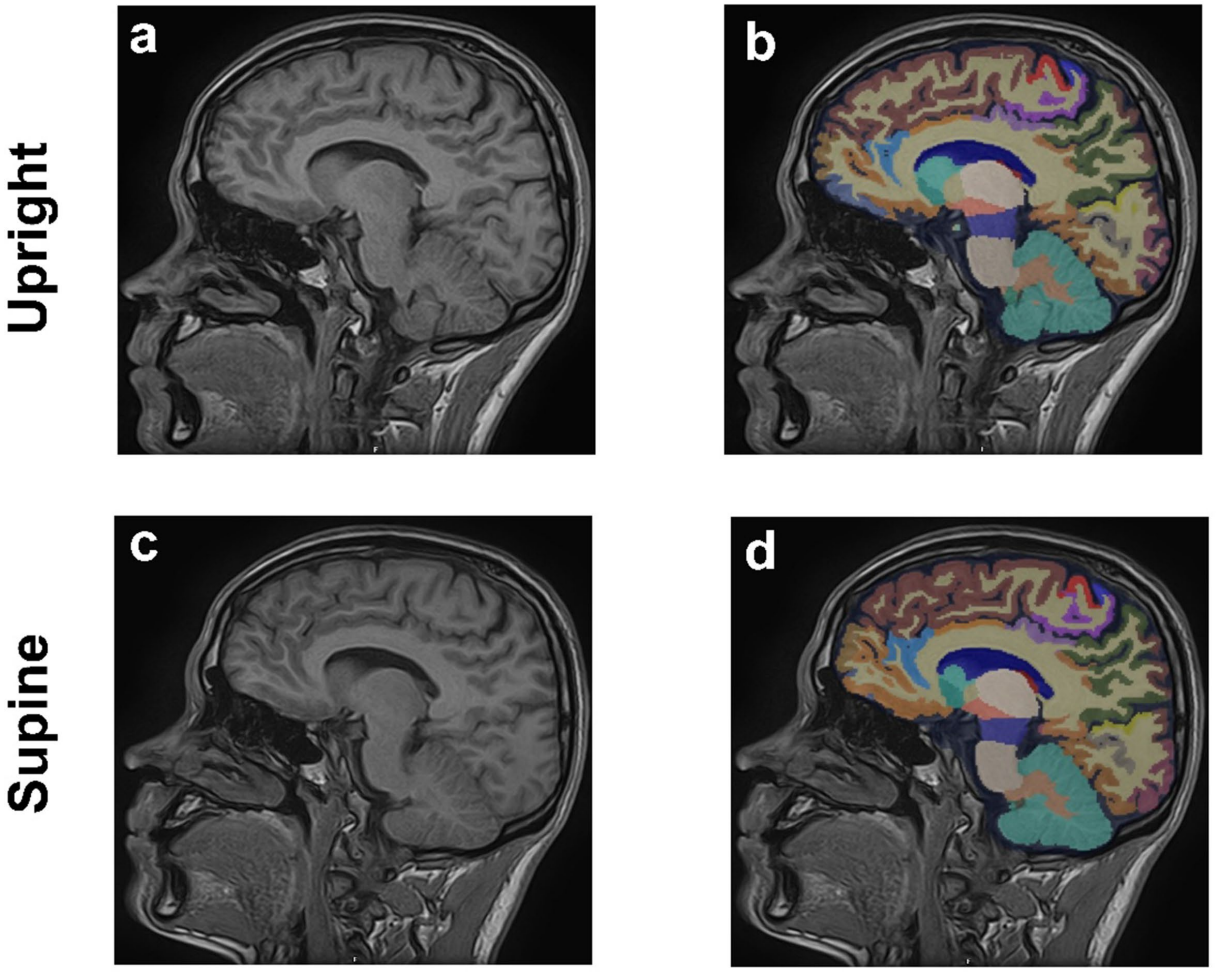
Sagittal 3D T1-weighted MPRAGE MRI images of the brain from a 29-year-old male in upright (a,b) and supine (c,d) positions. Anatomical images are shown in (a,c), (b,d) corresponding regional brain segmentations.
